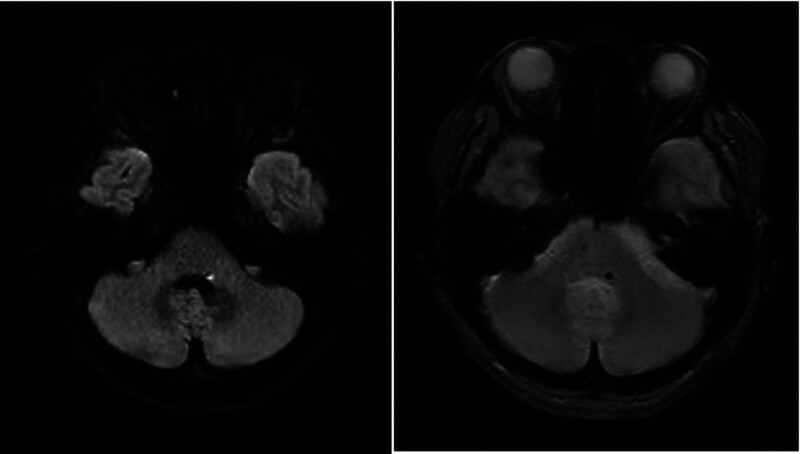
Patient brain magnetic resonance imaging. Diffusion-weighted imaging revealed a hyperintense lesion on the left dorsal pons, and a T2*-weighted image revealed a hypointense signal in the corresponding area.
